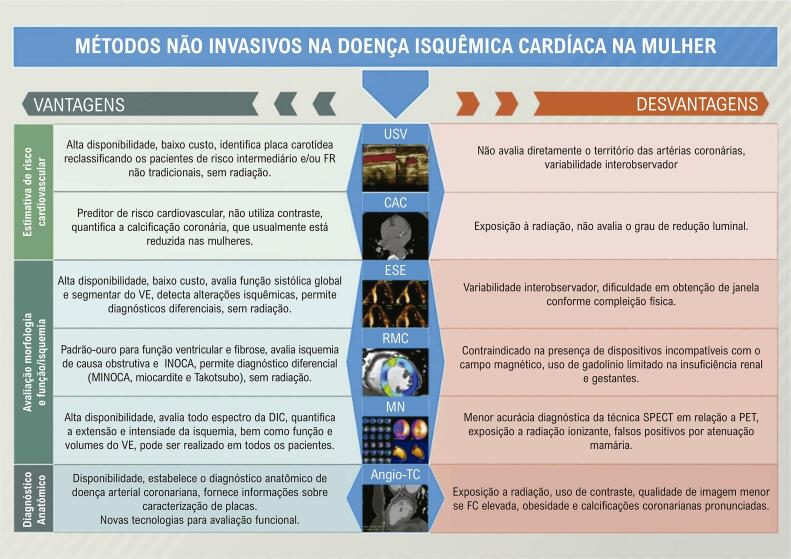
Importância dos métodos de imagem não invasivos na doença isquêmica cardíaca na mulher. Angio-TC: angiotomografia computadorizada de coronárias; CAC: escore de cálcio; DIC: doença isquêmica cardíaca; ESE: ecocardiograma sob estresse; FC: frequência cardíaca; FR: fator de risco; INOCA: isquemia na ausência de obstrução arterial coronariana; MINOCA: infarto do miocárdio na ausência de obstrução arterial coronária; MN: medicina nuclear; PET: tomografia por emissão de pósitrons; RMC: ressonância magnética cardíaca; SPECT: tomografia computadorizada por emissão de fóton único; USV: ultrassom vascular (carótidas); VE: ventrículo esquerdo.
